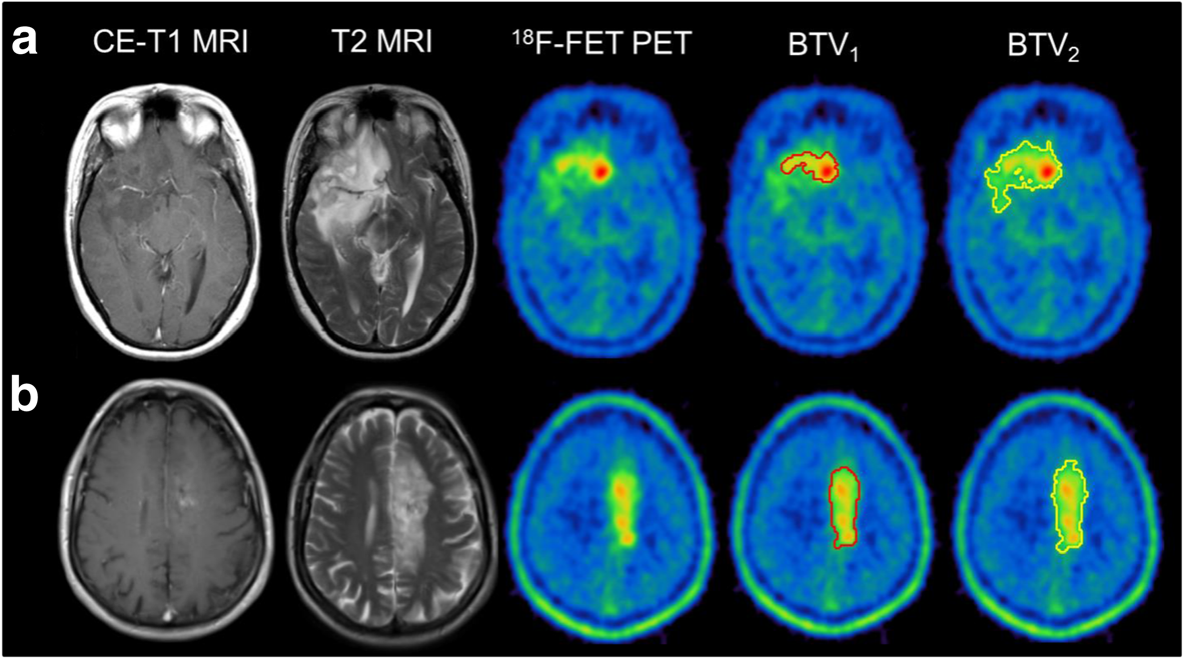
Influence of different background activity values on TBR_max_ and BTV values. a An example of a simple 2D-ROI assessment with measured background SUV values between 1.17–1.50: the resulting TBR_max_ ranges between 3.10 and 4.01 (ΔTBR_max_, 0.91; %ΔTBR, 29%), the BTV delineated with the calculated lowest threshold of SUV 1.87 is 45.89 ml, while BTV using the threshold with the highest background SUV (threshold SUV 2.40) results in a BTV with 9.91 ml only (ΔBTV, 35.98 ml; %ΔBTV, 78%). b The guided VOI assessment with a maximal background SUV range of 0.83–0.87 results in a small TBR range of 2.99–3.13 (ΔTBR_max_, 0.14; %ΔTBR_max_, 4%) and a BTV range of 20.53–22.63 ml (ΔBTV, 2.10 ml; %ΔBTV, 9%)
